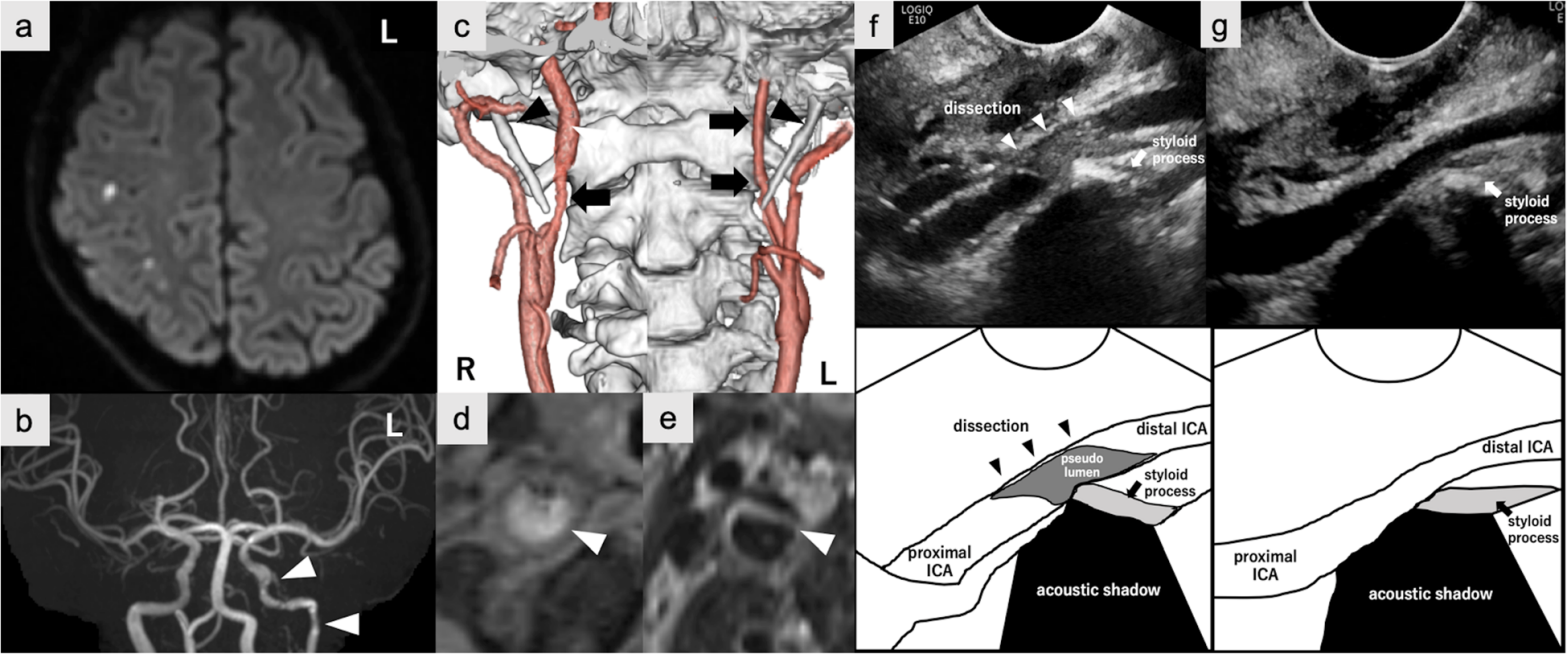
Diagnostic findings of bilateral ICA dissection and TOCU findings of right ICA dissection. a: Axial diffusion-weighted imaging showing multiple hyperintense signals in the right middle cerebral artery territory. b: Intracranial MRA showing no occlusion or stenoses in the right ICA territory. Multifocal segmental left ICA narrowing indicating previous arterial dissection *(arrowheads)*. c: Cervical CTA showing right extracranial ICA stenosis *(arrow)* and left ICA narrowing from the extracranial ICA *(arrows)*, accompanied by the bilateral elongated styloid process (*arrowheads*) close to each side of ICA. d and e: Cervical MRI showing intramural hematoma in T1-weighted imaging d and double-lumen in T2-weighted imaging e. f: Longitudinal view of TOCU showed right ICA stenosis with a thrombosed pseudolumen due to artery dissection (*arrowheads*). The styloid process (*arrow*) is spatially close to the dissection site accompanied by an acoustic shadow. g: Right ICA stenosis with a thrombosed pseudolumen decreased for 4 months of follow-up
